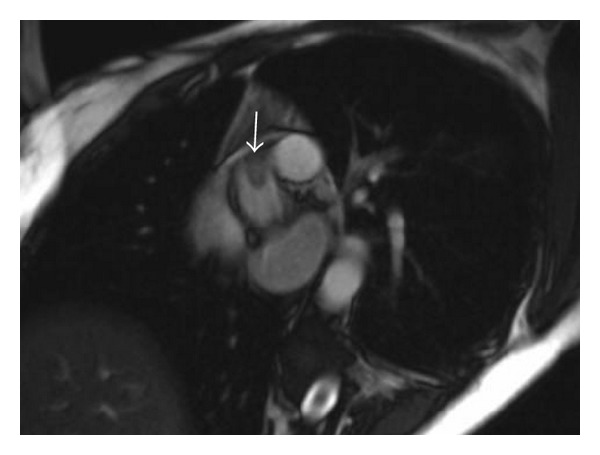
MRI image showing that there is a 1.3 cm pedunculated mass that arises from the right coronary * *cusp of the aortic valve. This mass also demonstrates enhancement on late * *gadolinium-enhanced images and is hyperintense on fat sequences consistent with papillary fibroelastoma.
